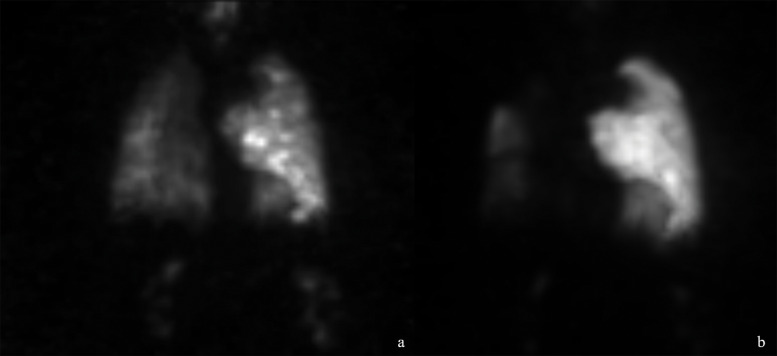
Coronal SPECT-scan taken three weeks after the initial presentation. (a) Ventilation imaging showing globally reduced tracer uptake in the right lung without distinct ventilation defects. (b) Perfusion imaging showing near-complete absence of right lung perfusion with minimal anterior preservation and mildly heterogeneous left lung perfusion without distinct defects.
